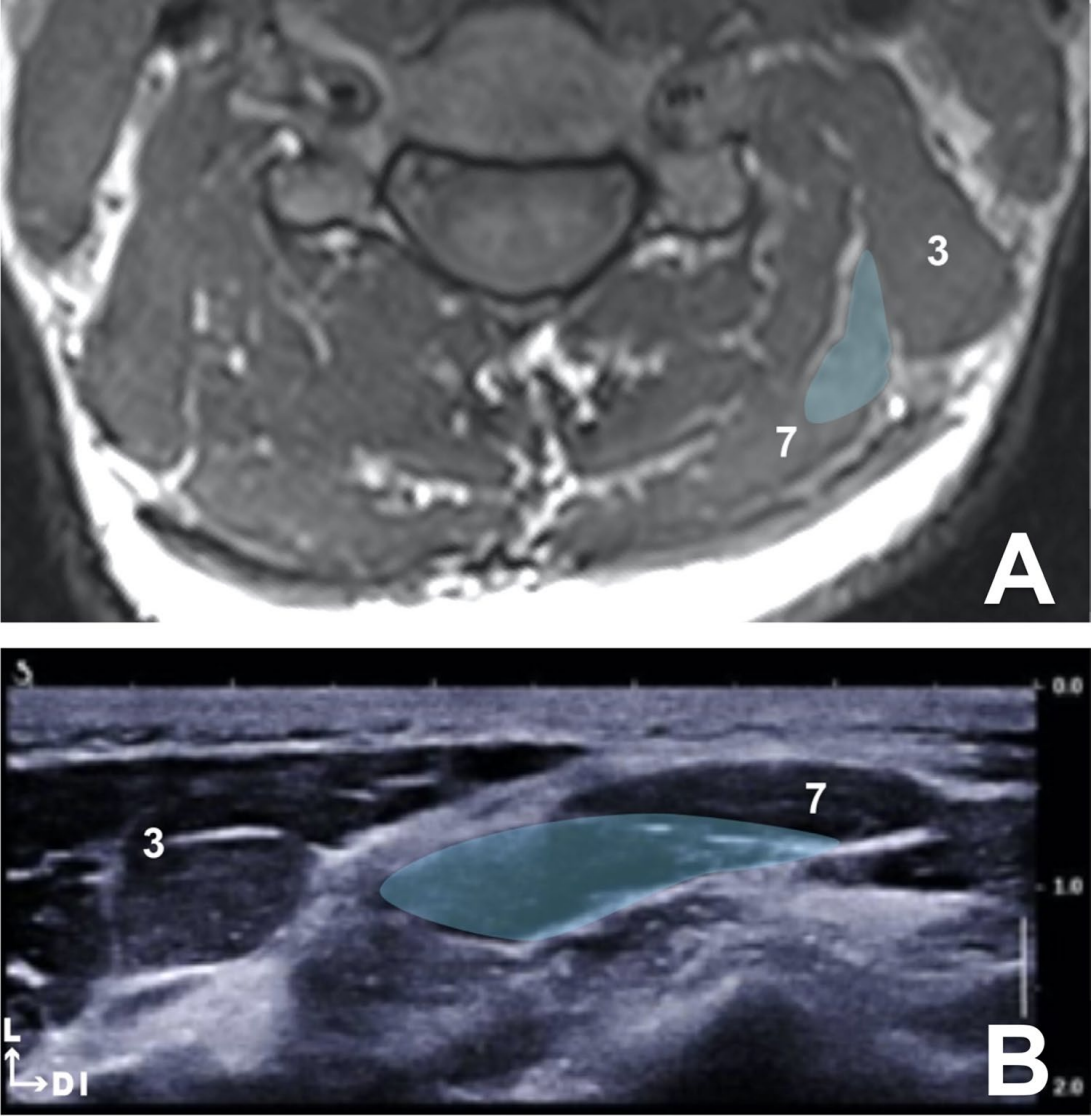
a T1-weighted MRI axial slice at the C5 vertebrae level. Splenius cervicis (blue). Levator scapulae (3) and splenius capitis (7). b Ultrasound image between C4 and C5 spinous processes. Splenius cervicis (blue). Levator scapulae (3) and splenius capitis (7)
